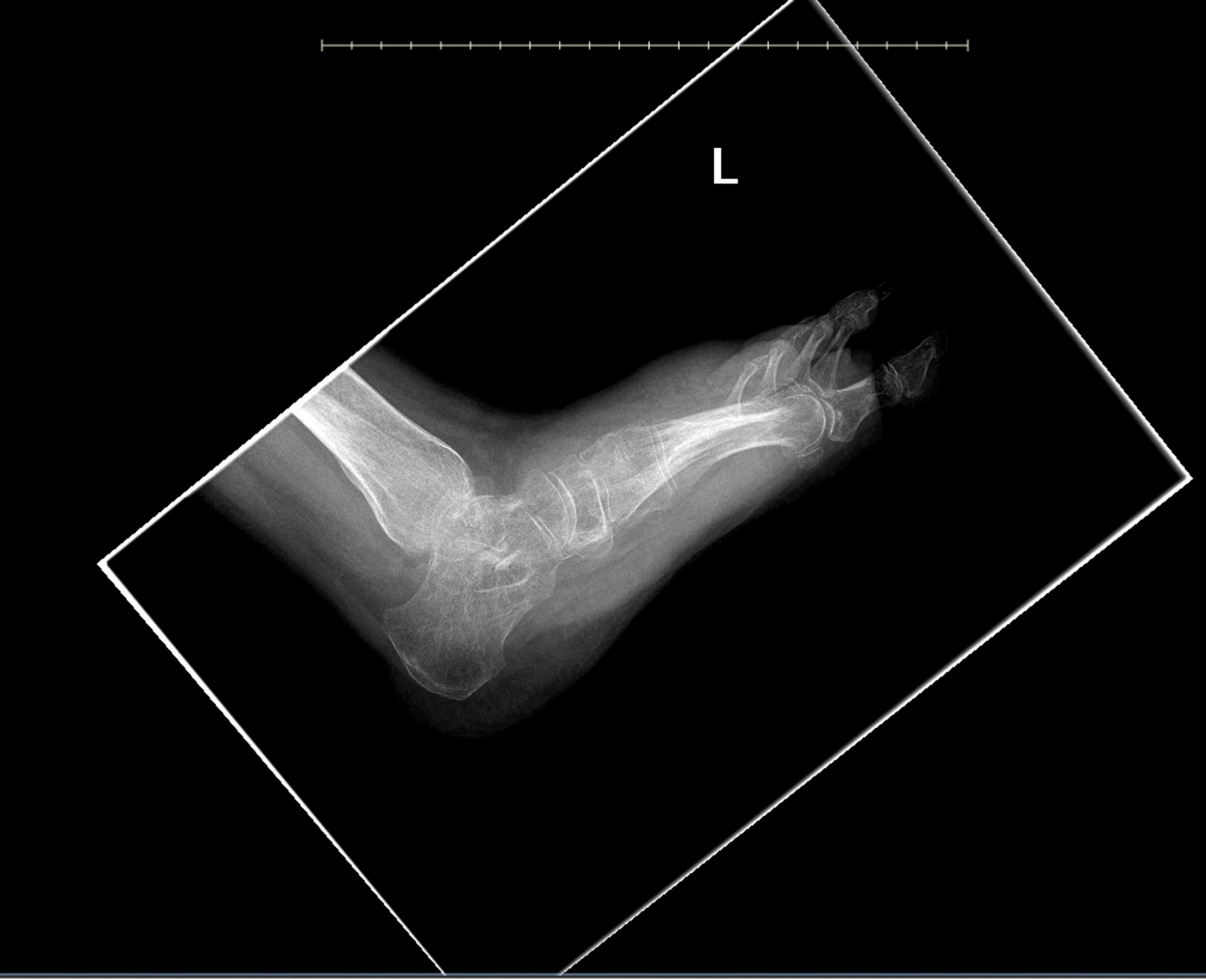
Left foot imaging from February 2025 Image impression shows no acute fracture or dislocation. Chronic fracture third metatarsal base. Osseous demineralization. No radiographic evidence of osteomyelitis. A large amount of soft tissue swelling
